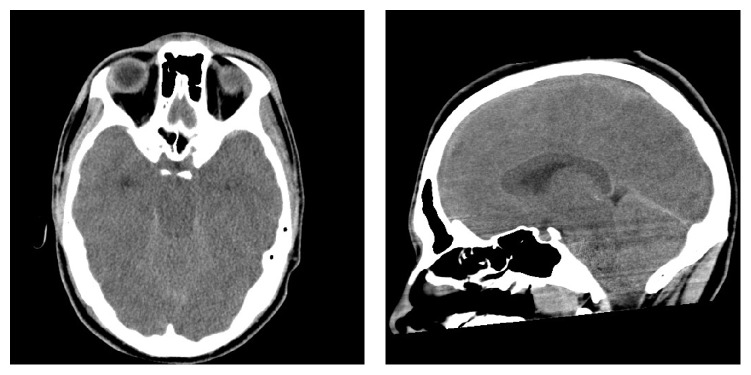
a (left): brain CT scan showing a diffuse edema with the disappearance of the gray/white matter limitation; b (right): herniation of the cerebellar tonsils.
